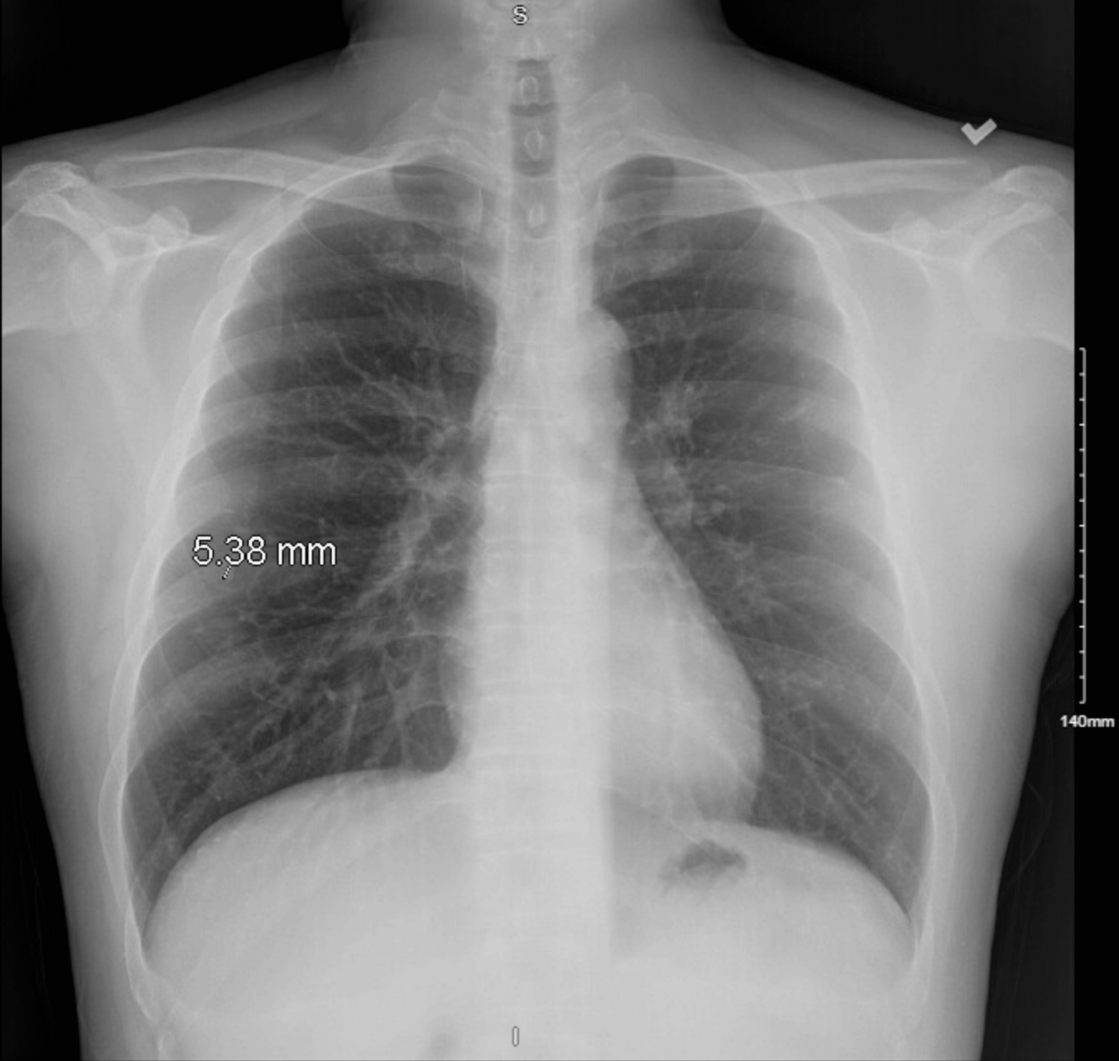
Initial chest X-ray demonstrating a 5.38 mm nodular density projected over the posterior aspect of the right 8th rib. While this may represent a vessel seen end-on, the size is slightly prominent for the location, and a small pulmonary nodule cannot be excluded.
